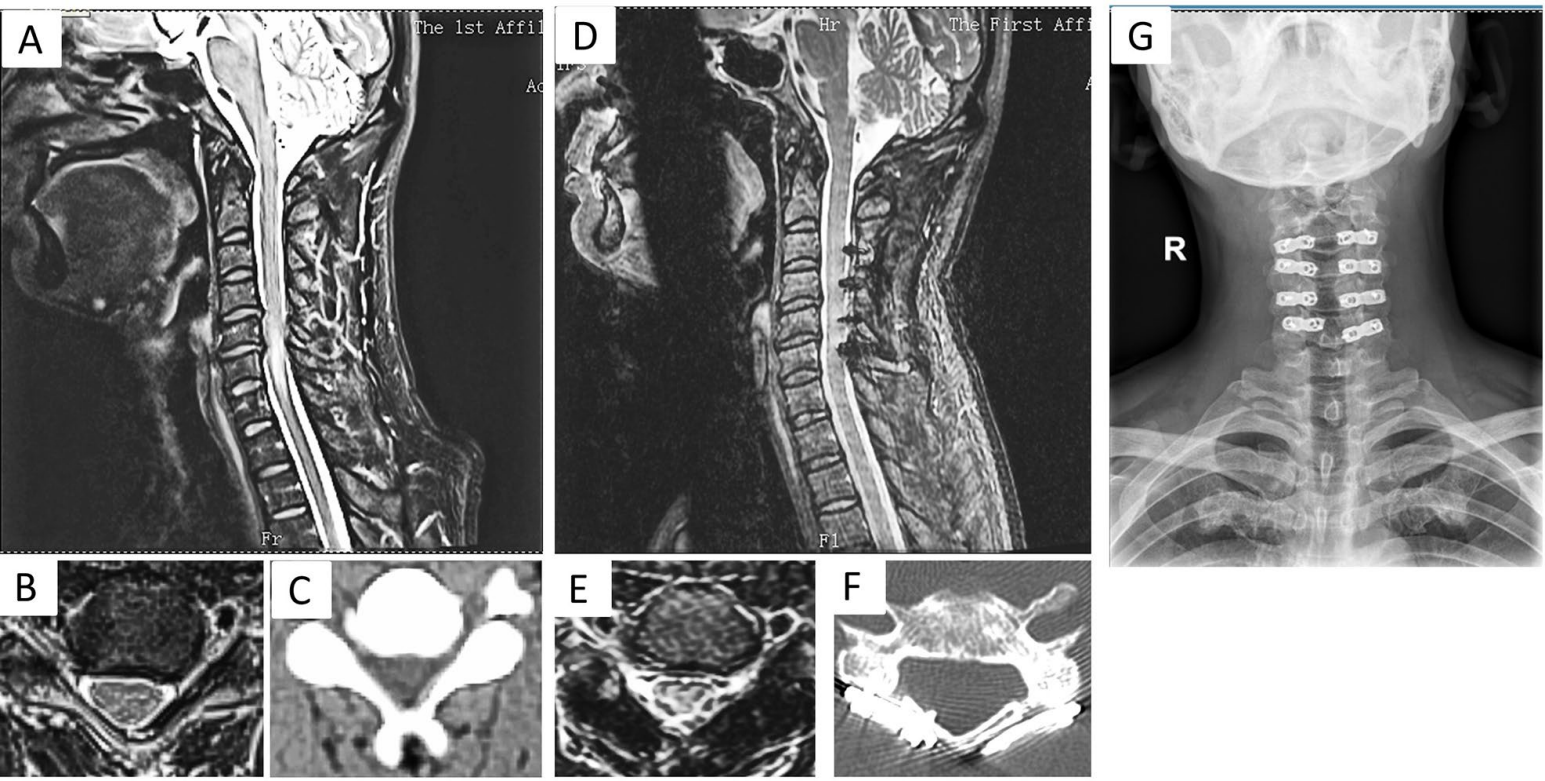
Preoperative and postoperative MRI results showing a spontaneous regression of CDH in one patient with two-level myelopathy. The represented MRI image on sagittal view, coronal view, and CT scan results pre-operatively (A–C) and two years post-operatively (D–F) demonstrated the resolution of CDH after CMEL (regress ratio of 34.9% and 34.4% for C4/5 and C5/6). (G) Postoperative X-ray images showed the cervical spine morphology after CMEL.
